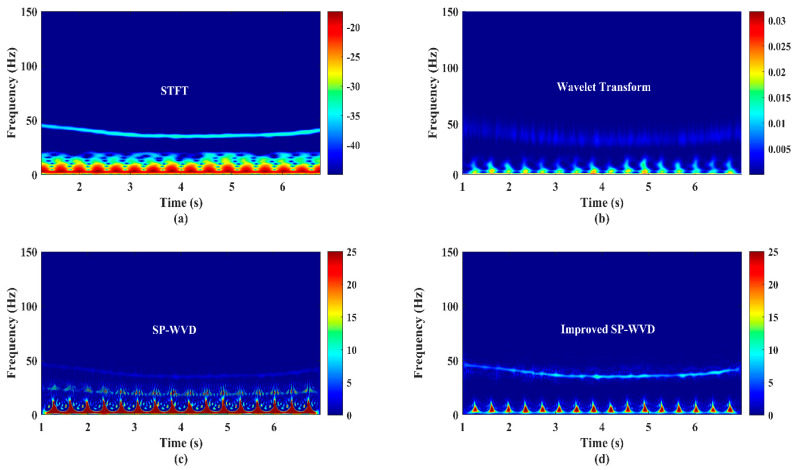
The bionic bird’s sustained−flight action’s and a three−blade rotational propeller system’s micro−Doppler signature/pattern image designed using (a) short−term Fourier transform (STFT), (b) wavelet transform, (c) SP−WVD and, and (d) improved SP−WVD.
